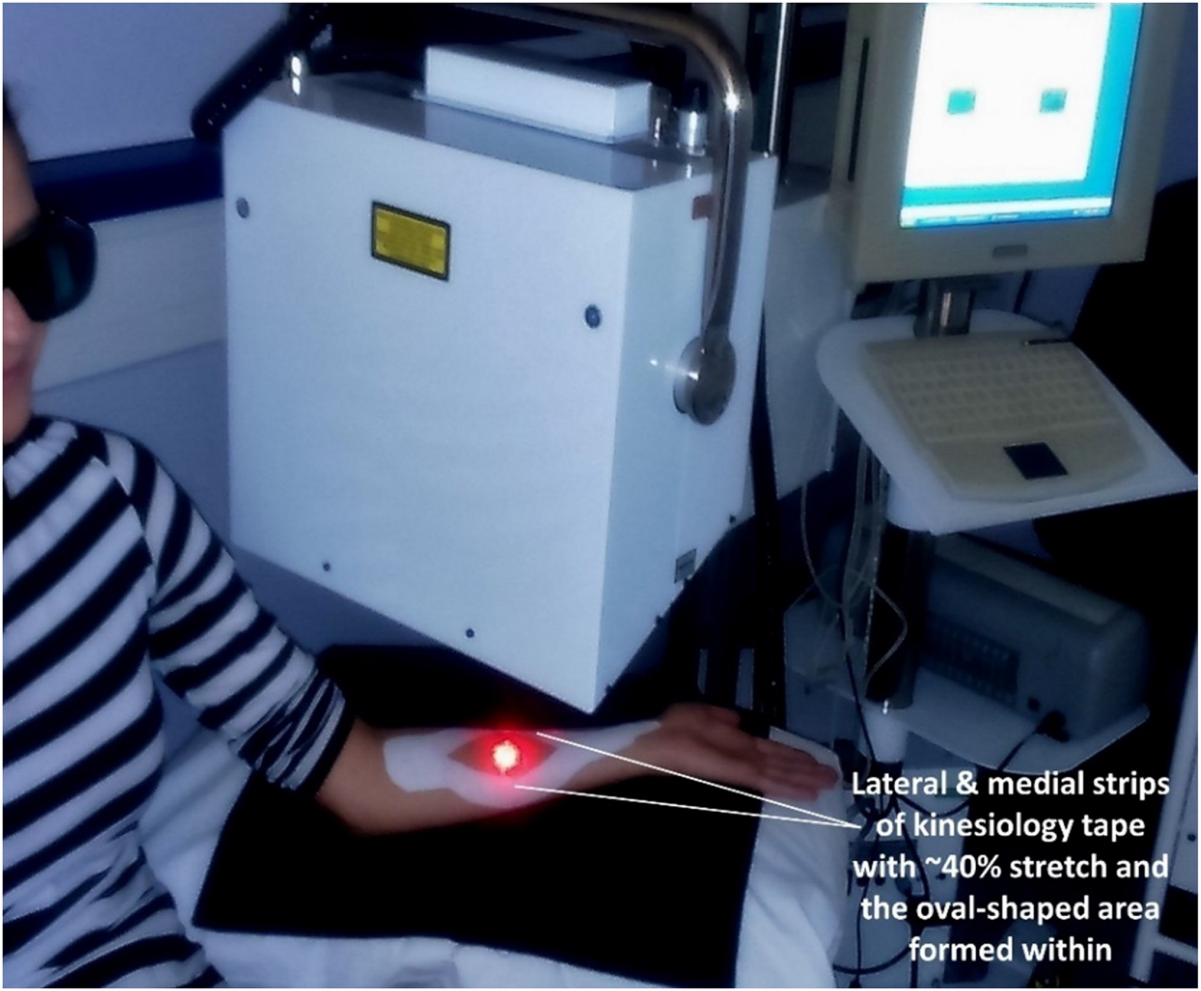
Demonstration of laser Doppler imaging of the oval-shaped site of testing formed by the inner margins of lateral and medial strips of tape.
